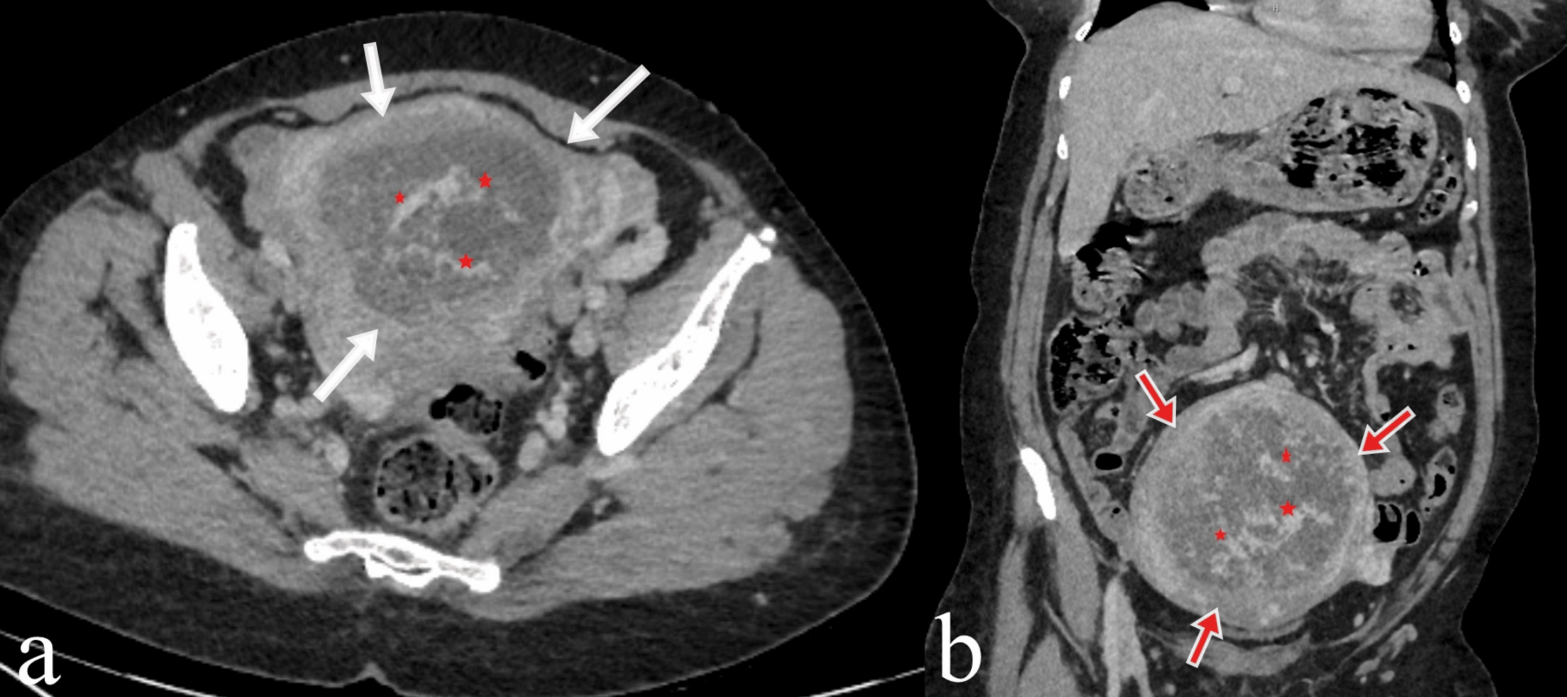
Axial and coronal contrast-enhanced abdominopelvic computed tomography images of the mass lesion (a) Axial and (b) coronal contrast-enhanced abdominopelvic computed tomography images show a heterogeneous mass lesion in the uterus (white and red arrows). There is a more pronounced contrast enhancement in the center of the mass (red asterisk).
